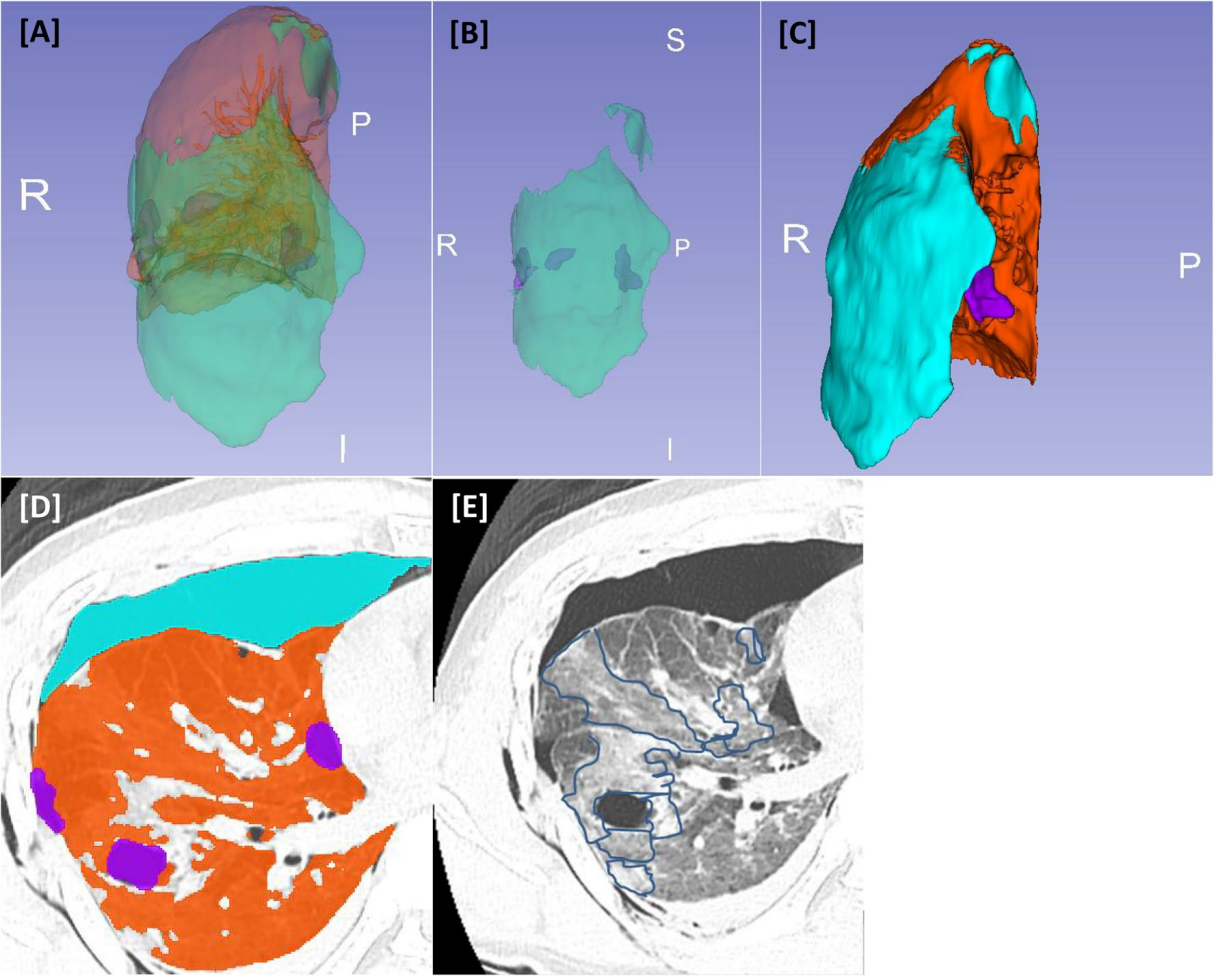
Process of lung segmentation with a three-dimensional (3D) slicer. The 3D model can be viewed after setting the most appropriate Hounsfield unit for lung segmentation. (A) anterior view, (B) parts excluding normal lung area, and (C) posterior view. Blue indicates the pneumothorax region, orange indicates the normal lung area, and purple indicates the segmented region with pulmonary contusion. (D) transverse view on chest computed tomography. (E) If there is an actual contusion and it is missing, it is indicated by hand labeling. *R* right, *P* posterior, *I* inferior.
